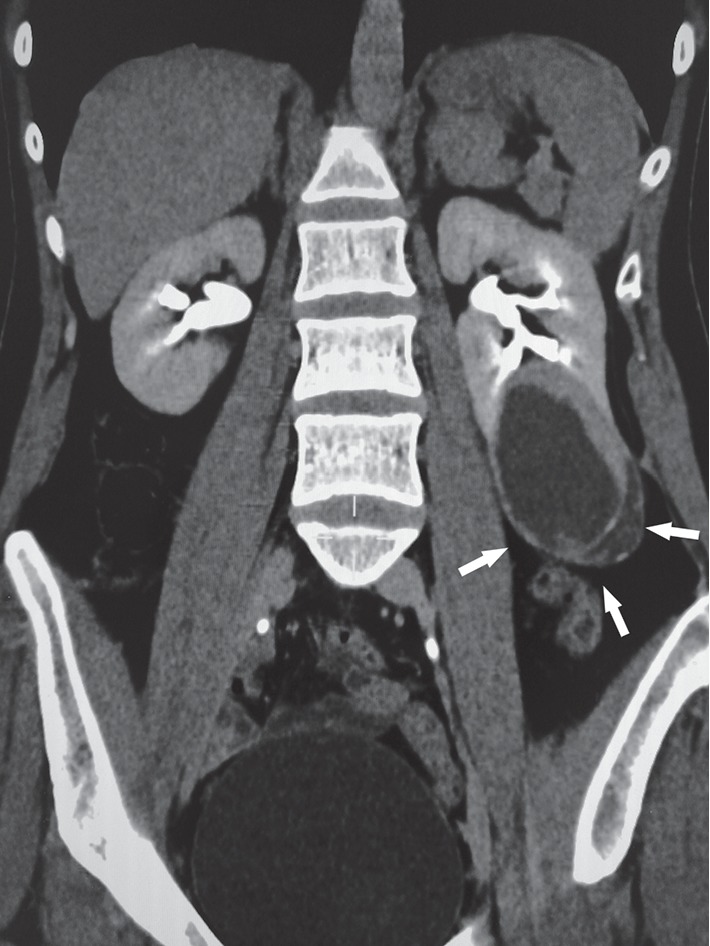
CT scan revealed that there was a cystic solid lesion at the lower pole of her left kidney, which protruded the renal parenchyma with a clear boundary (arrow).
